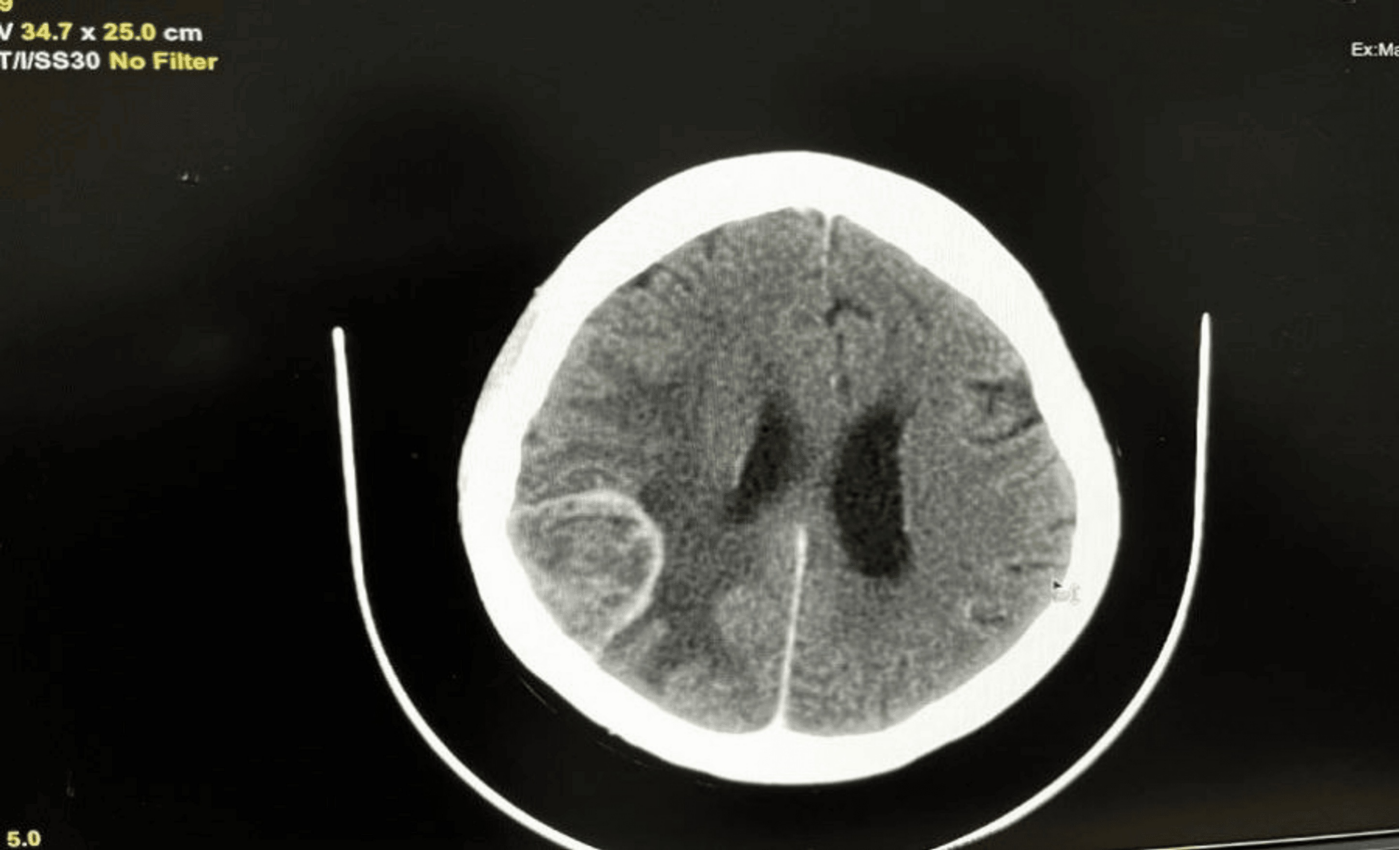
A heterogeneous enhancing solid mass lesion of size 41 x 30 mm in the right posterior parietal region with moderate surrounding edema suggestive of metastasis as observed via CECT CECT, contrast-enhanced computed tomography.
